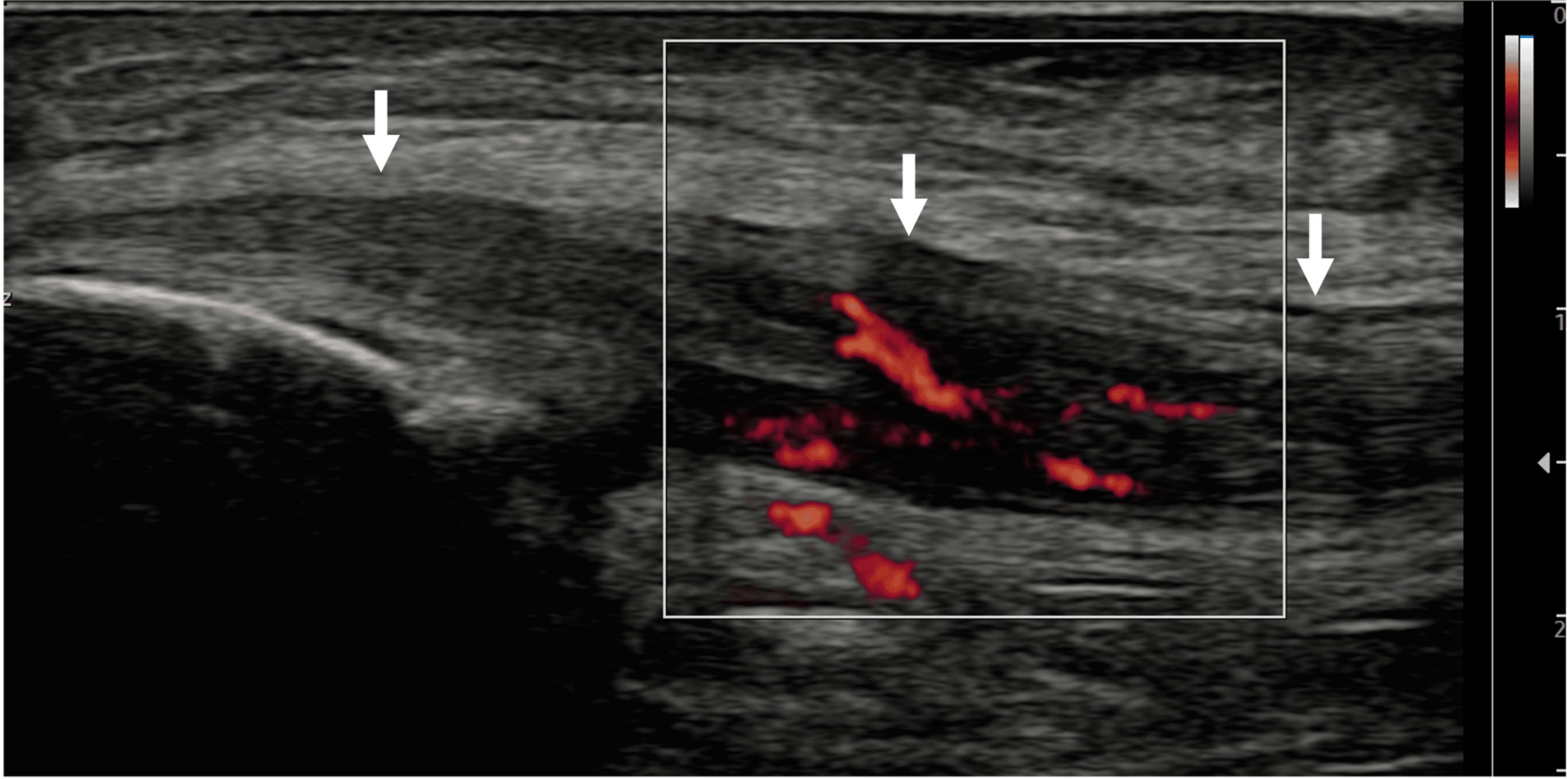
Long-axis view of the patellar tendon with MV-Flow tool showing hyperemia within the tendon. Arrows: Patellar tendon. NOTE: MRI images for this patient were not available for publication.
